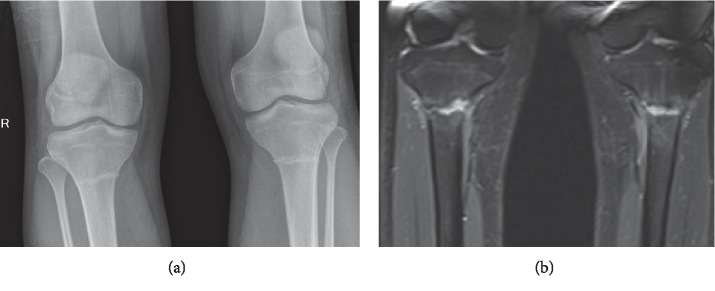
X-ray and MRI. (a) X-ray of the patient's legs showed cortical irregularity and lucency with sclerotic margins in the proximal shaft of the tibia, confirming stress fractures. (b) MRI of the calves of the patient showed increased signal at linear T1RM sequence which suggests fluid clefs at both fracture sites.
